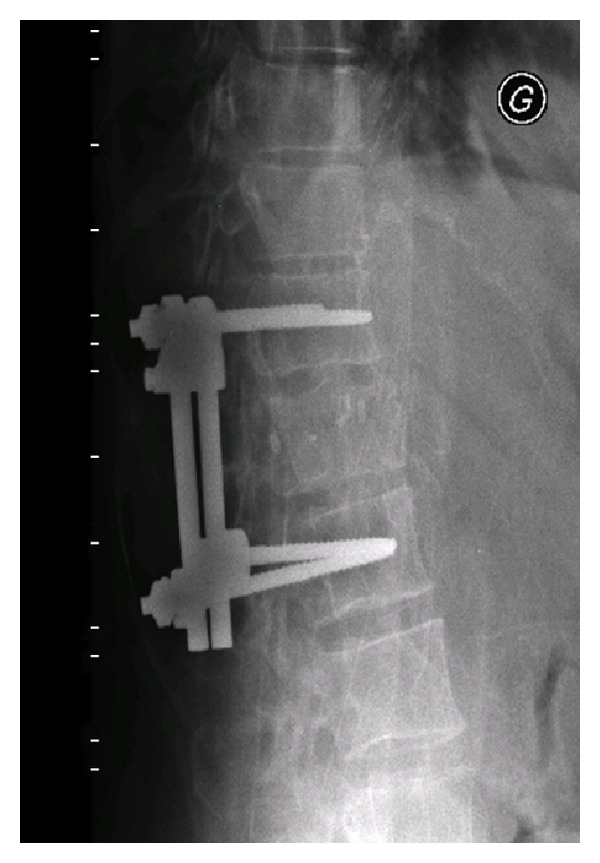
Postoperative lateral X-ray showing the height restitution of T12.
